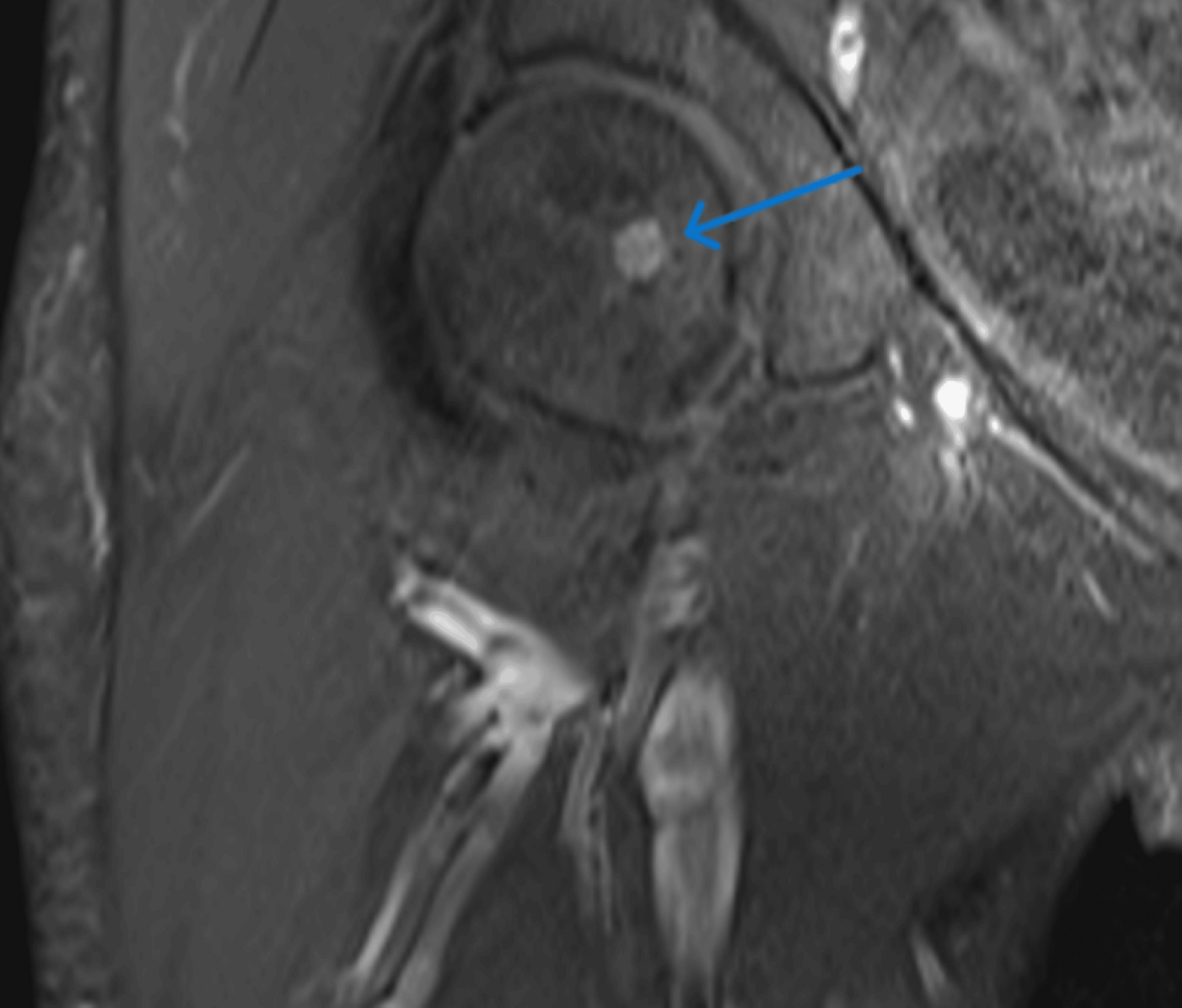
MRI of the hip showing a 5-mm enhancing lesion at the right femoral head
